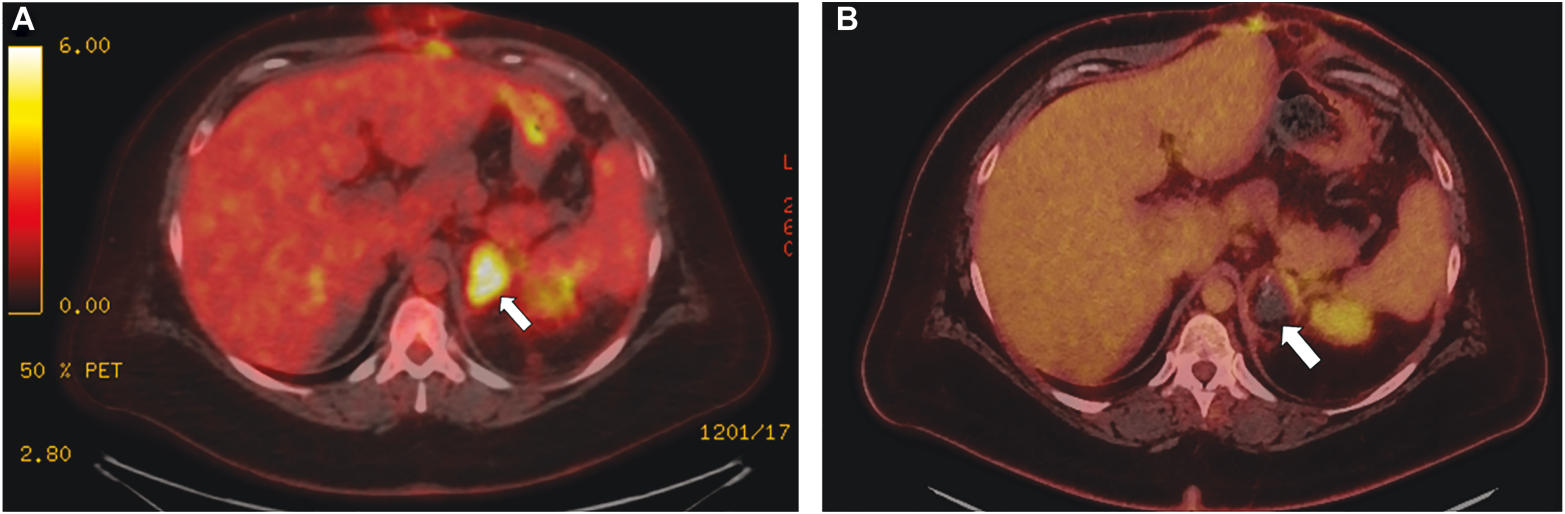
Positron emission tomography scans of a patient who was diagnosed with left adrenal adrenocortical carcinoma. (A) An FDG-avid tumor in the left adrenal gland was resected using left open adrenalectomy with R2 resection and shows FDG uptake in the left adrenal remnants. (B) Subsequent 3-month follow-up demonstrates no FDG uptake, showing complete response to cryoablation.
